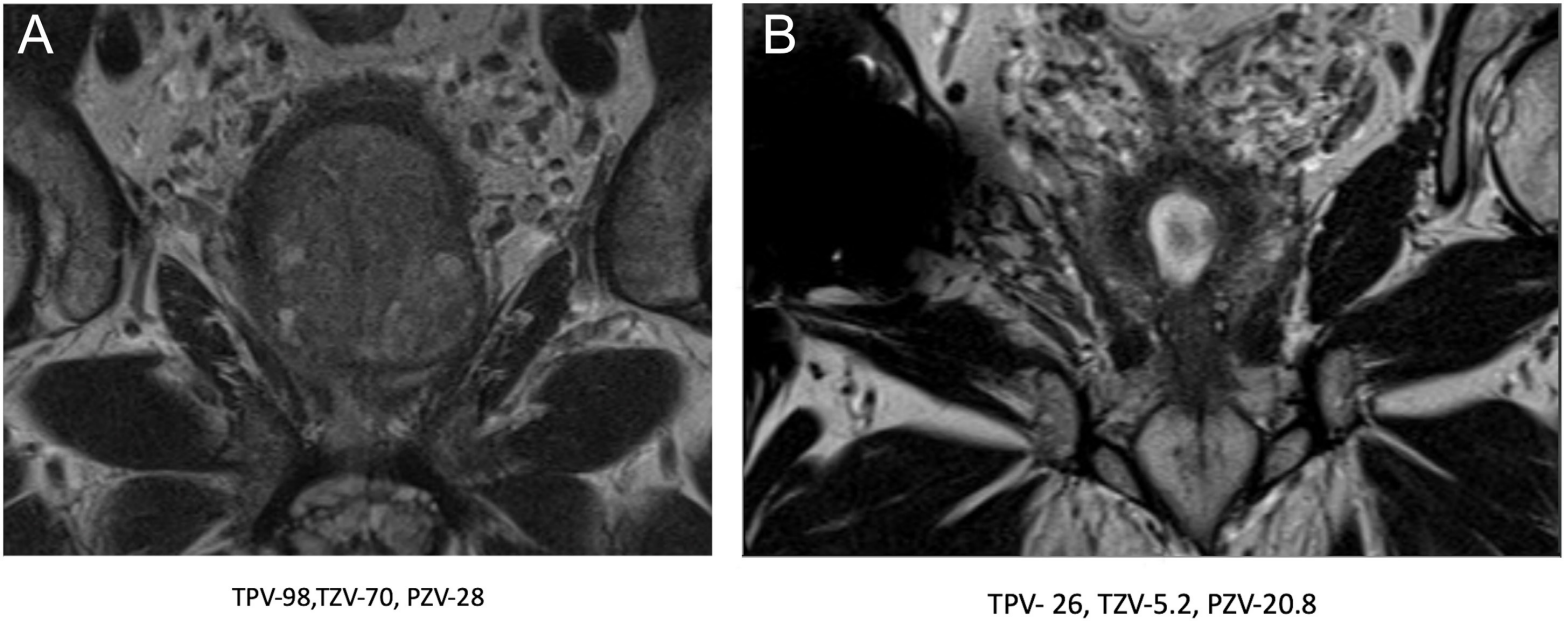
a,b. T2 weighted coronal MRI images before and 7 month after HoLEP procedure (volume in cc).
